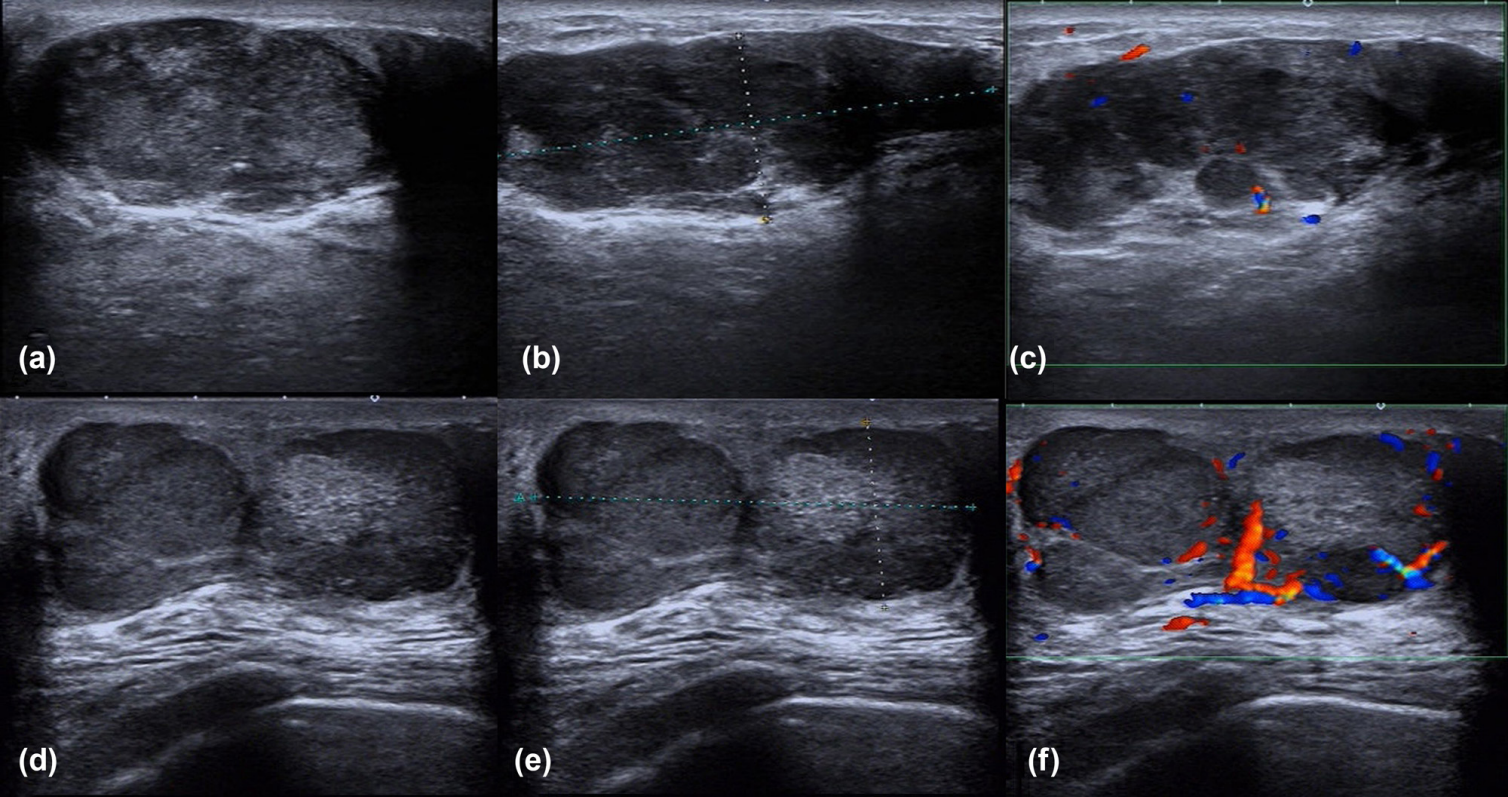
B-ultrasound images before treatment: This shows irregular hypoechoic clusters behind the left papillae, with irregular edges and uneven internal echoes, with parts of the area crisscrossing the surrounding tissue in a canine interlacing pattern, and no changes in echoes seen behind the multiple internal calcification points (a and b). CDFI: low echo mass and short rod-shaped blood flow signal within it (c). Following treatment, a reduction in the irregular hypoechoic mass was observed, with lobular structure and clear boundaries with surrounding tissue (d and e), CDFI: abundant blood flow signals in the hypoechoic mass (f).
